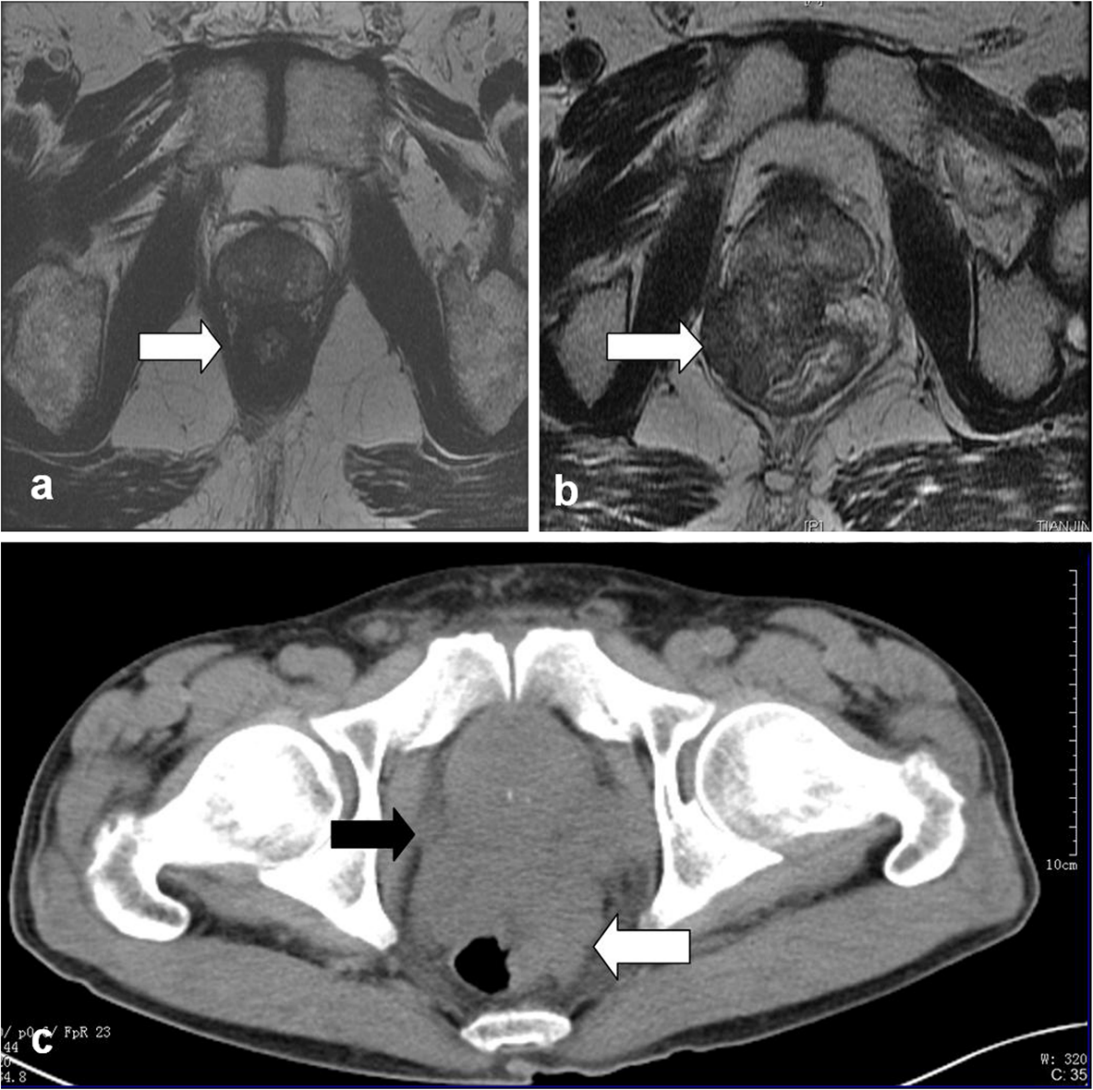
Typical images show prostate cancer invading rectum. a. Pelvic MRI showed a circumferential thickening of the rectum, although there was no continuity between prostate and rectum; b. Pelvic MRI showed a protruding anterior rectal mass which compressed the rectal lumen and continuity between prostate and rectum; c. CT of the abdomen and pelvis showed an irregular thickening of the rectum and continuity between prostate and rectum.
